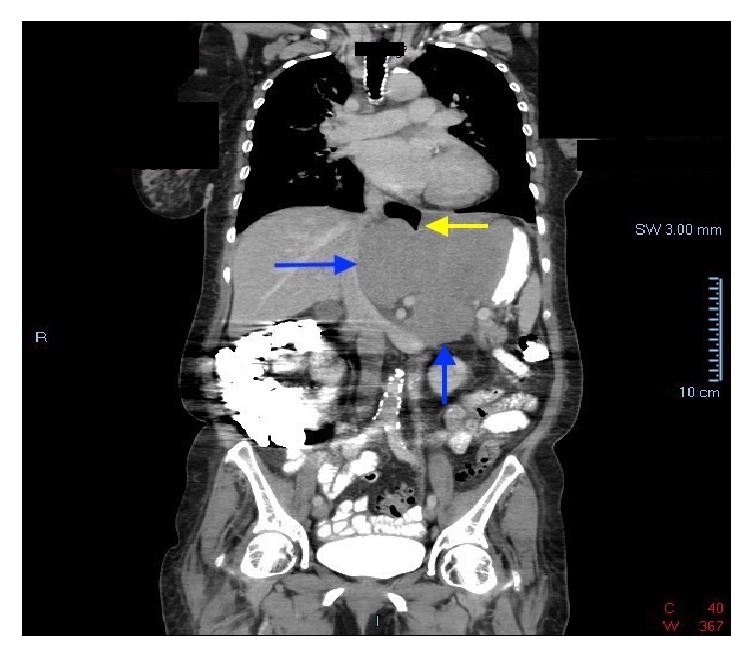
CT scan (coronal view) showing a 12 x 12 soft tissue mass in the gastrohepatic omentum (blue arrows) compressing the distal esophagus (yellow arrow) and gastric fundus.
